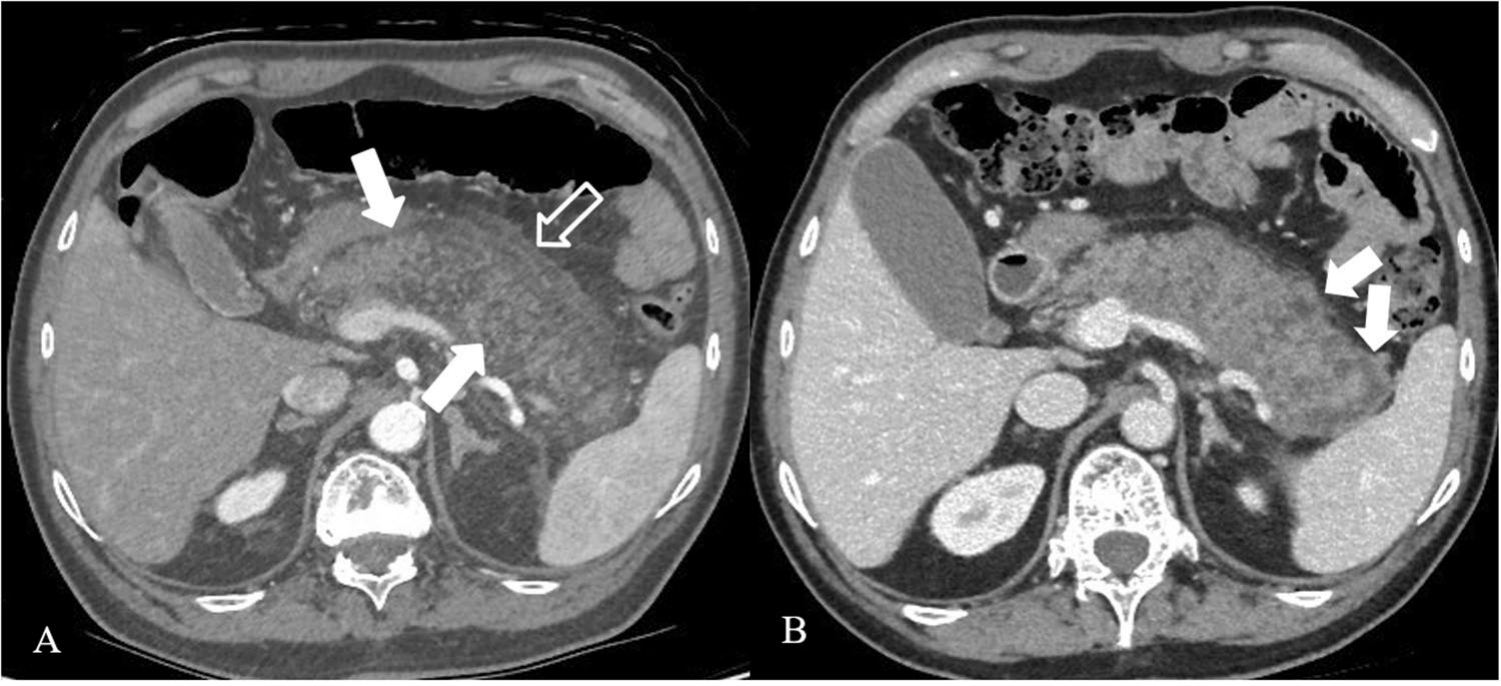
Acute pancreatitis in a 61 years-old man. The axial contrast-enhanced CT scan after 3 days from the onset (a), in pancreatic arterial phase, shows edematous pancreas (arrows), with disomogeneous and reduced enhancement, with no necrotic area in the contest. It is also evident mild peripancreatic fatty stranding (empty arrow). TC study after nine days of the onset (b) shows heterogeneous contrast enhancement of the pancreatic parenchyma, with focal hypovascular areas due to the necrosis (arrows)
